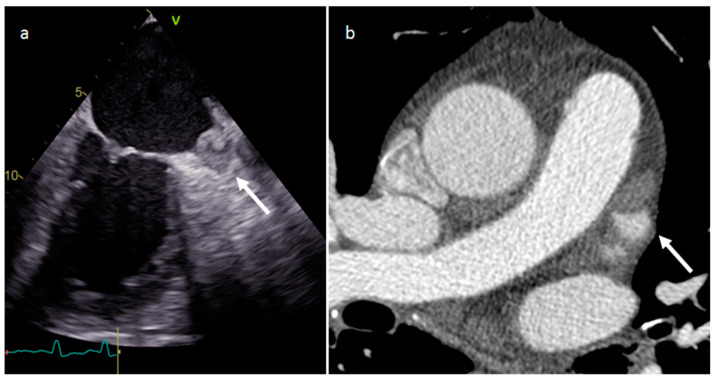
Left atrium appendage thrombus at transesophageal echocardiography (a) and CT (b).
